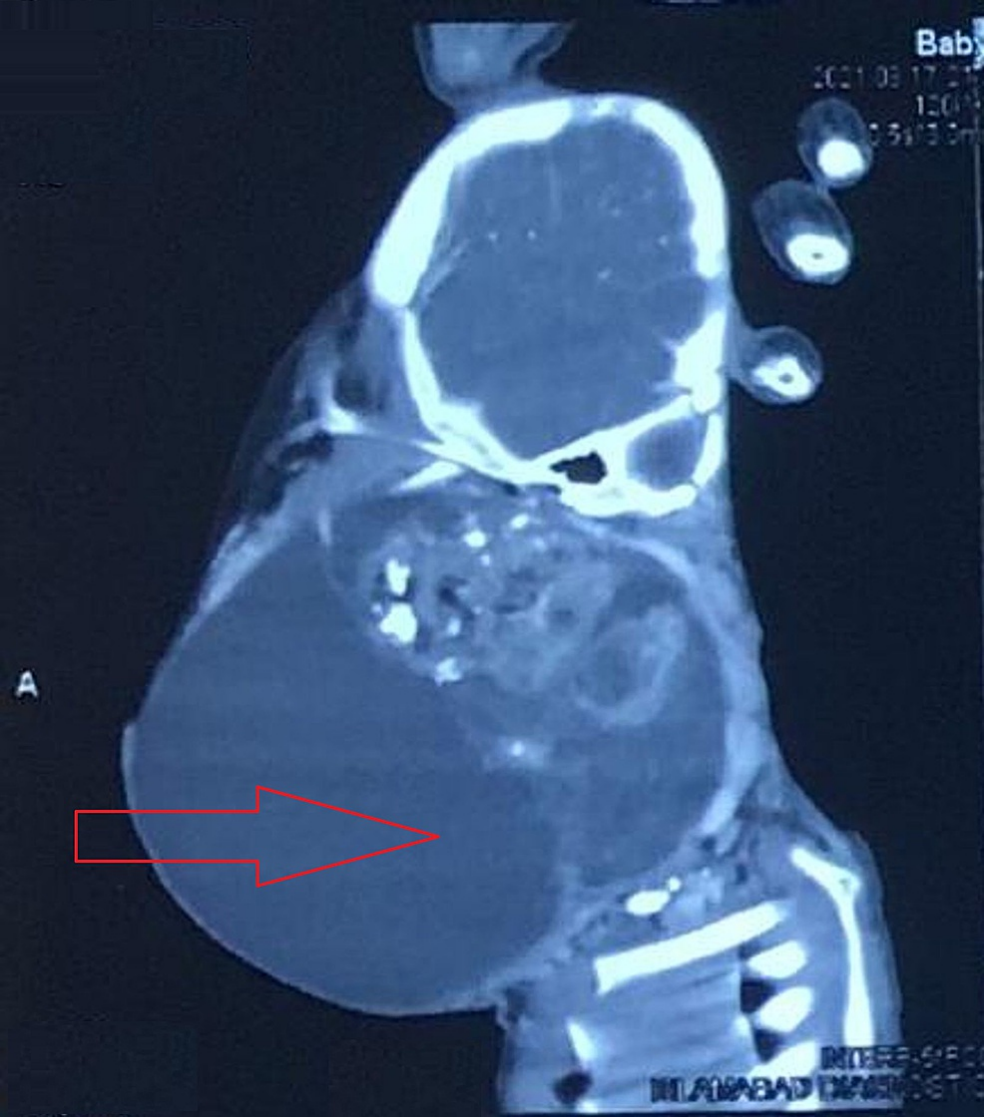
The CT scan of the head and neck shows a large exophytic mass with solid and cystic components on the left side of the neck as marked by the arrow. The mass is compressing adjacent structures.
